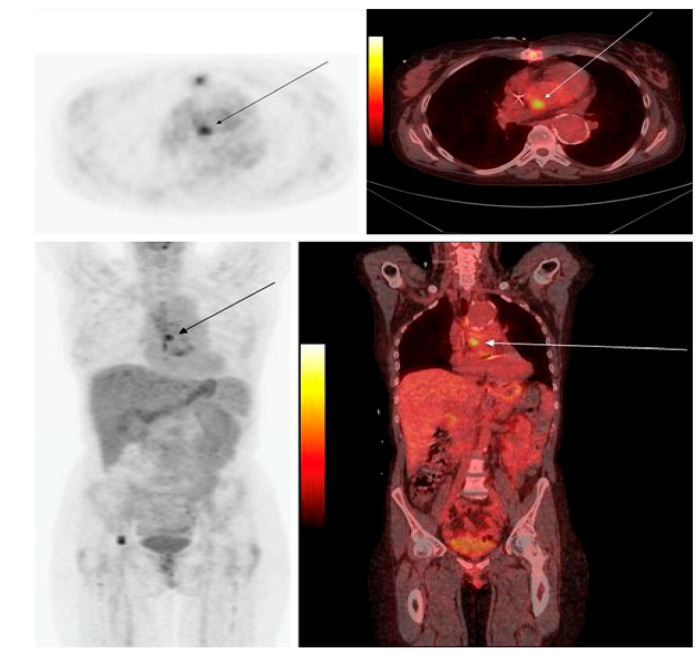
PET/CT in infective endocarditis. The PET/CT image of a 47-year-old female with IE: (Upper) transaxial image; (lower) coronal image, with PET on the left and the PET/CT fusion image on the right. The images depict a focal region of increased [^18^F]FDG uptake in the heart at the position of the valvula aorta (with permission from reference [34]).
